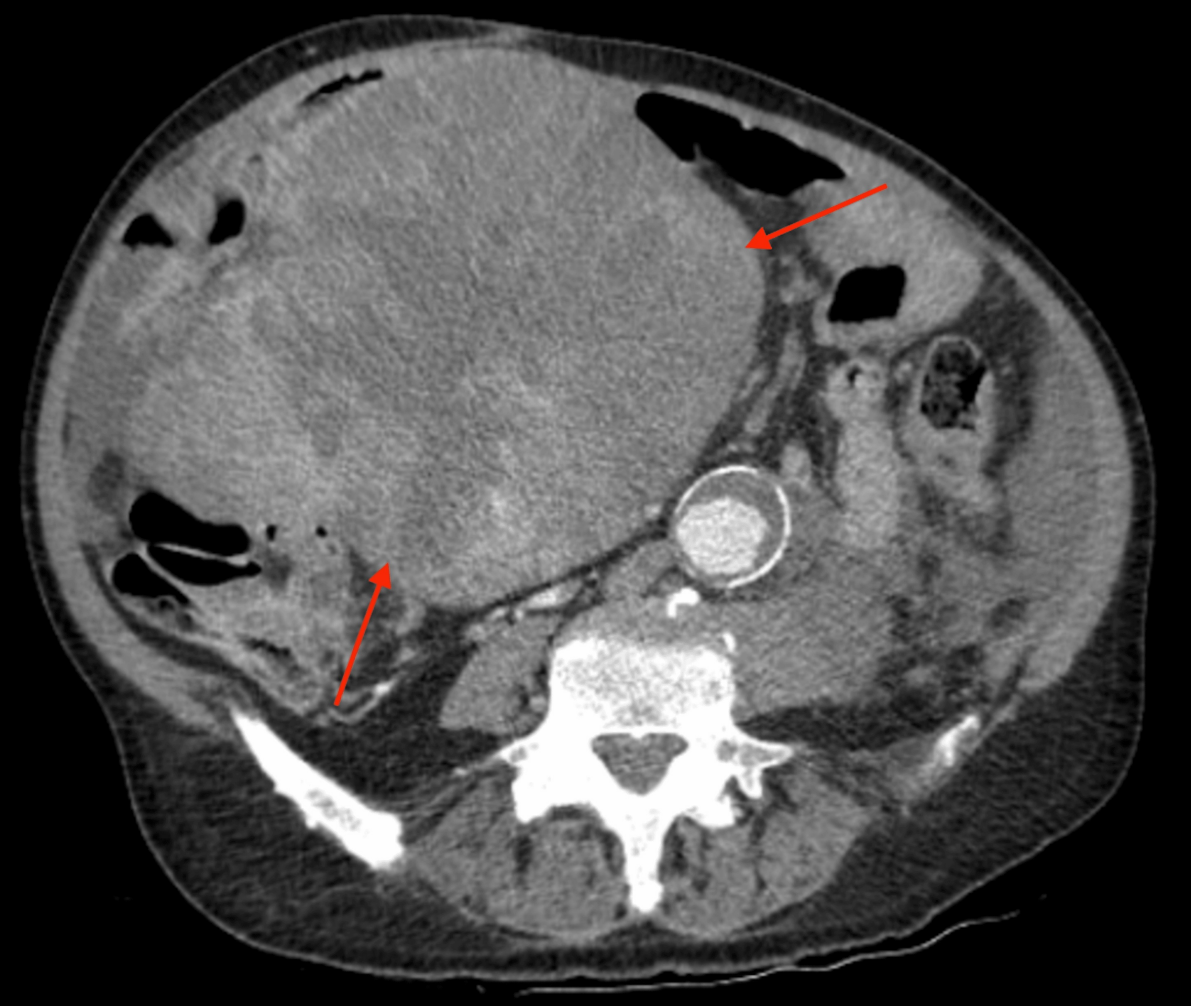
Axial CT view of the abdomen and pelvis, showing the large complex soft tissue mass (red arrows) extending from the right upper quadrant into the lower abdomen CT: computed tomography
